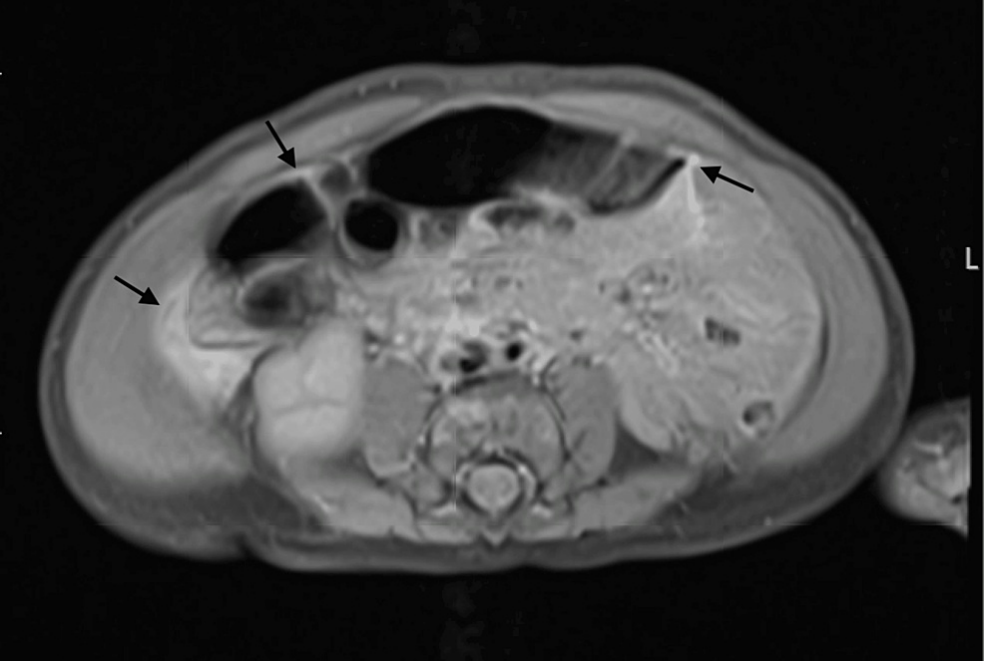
Trace chylous ascites on abdominopelvic magnetic resonance imaging (axial view). Arrows indicate areas with chylous ascites.
